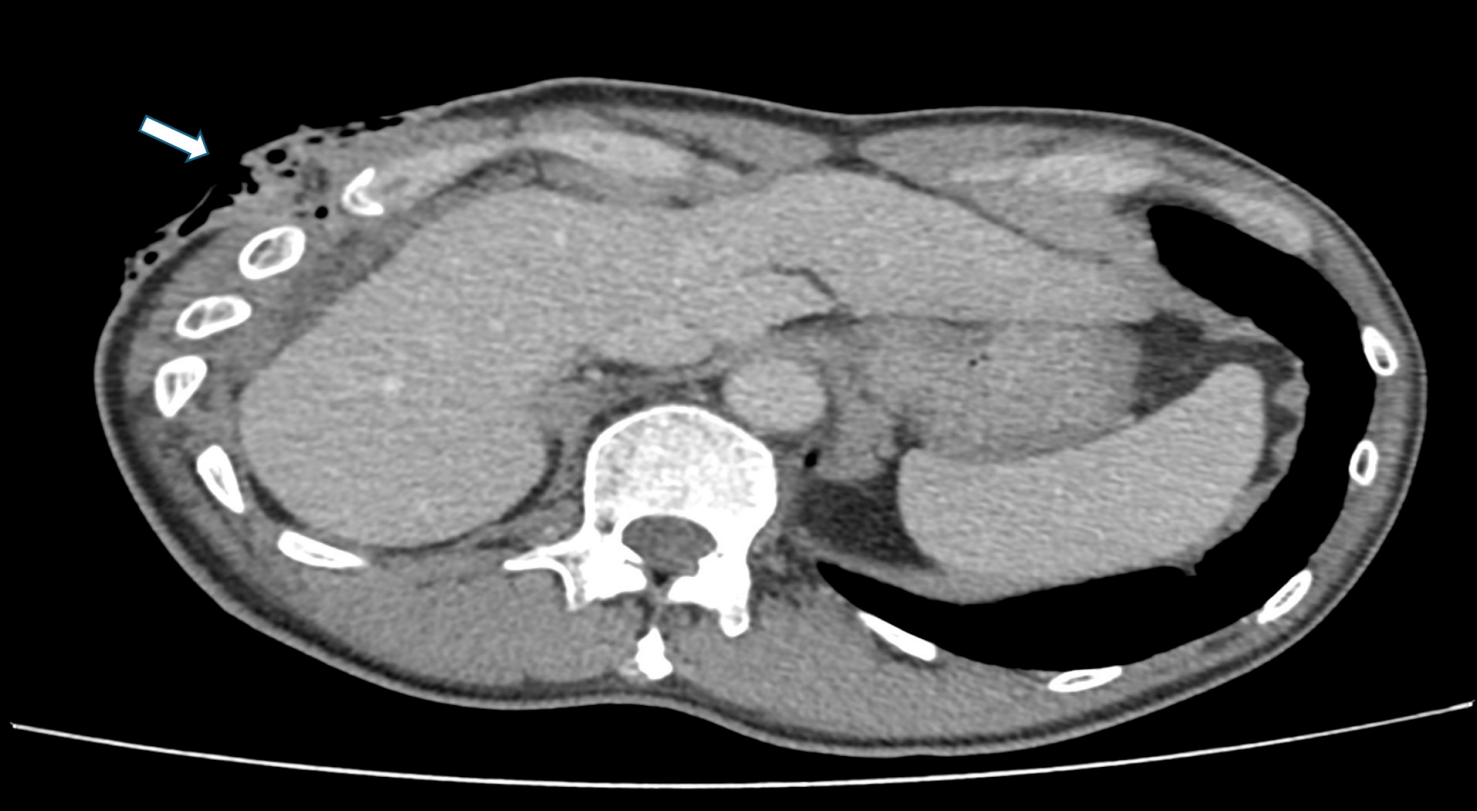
Axial CT depicting subcutaneous collection breaching the right anterior chest wall (arrow).
